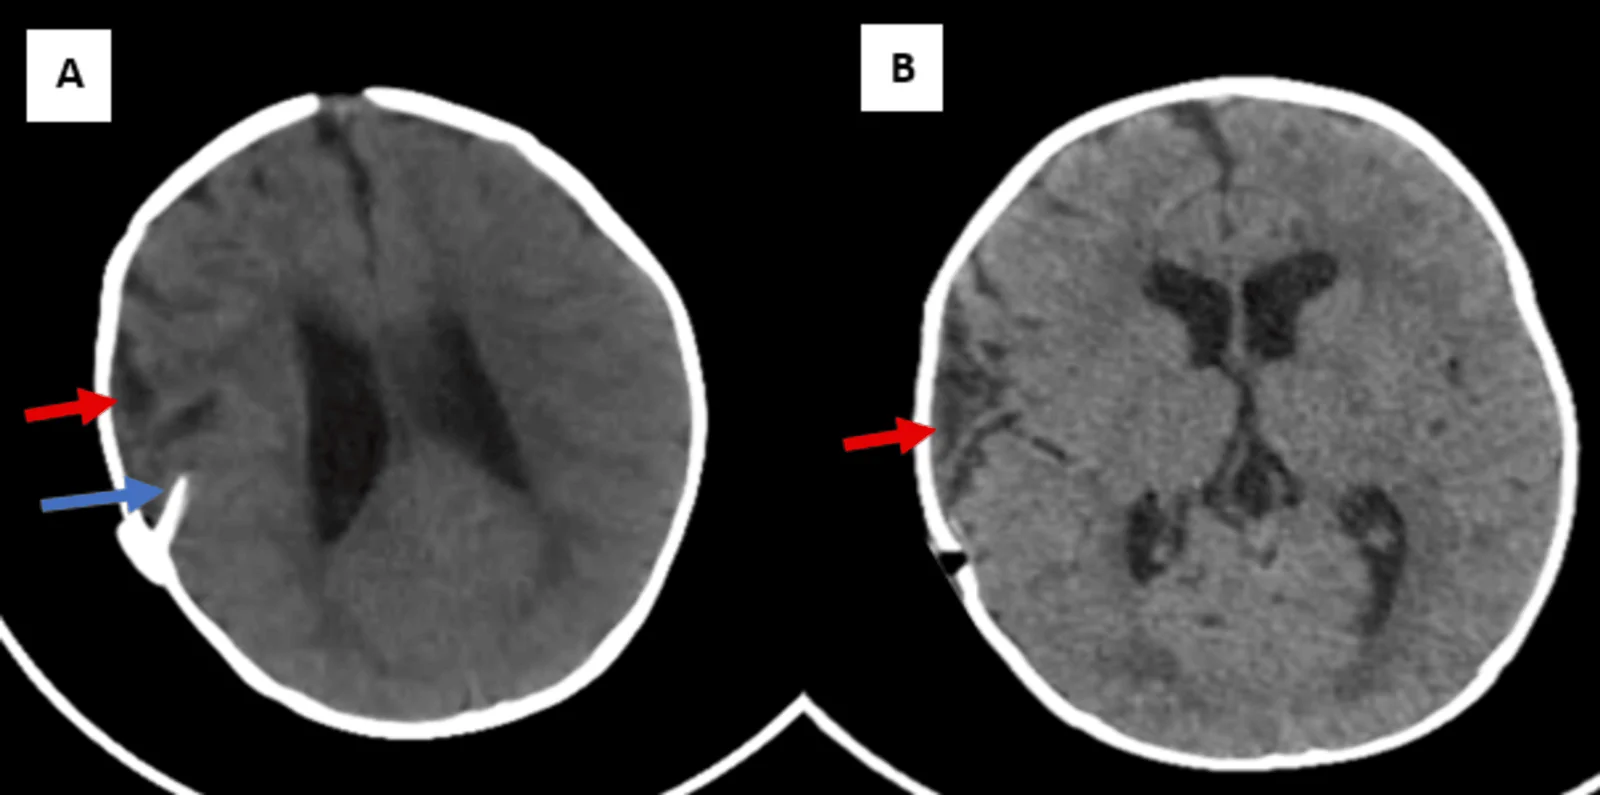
(A) Preoperative brain CT scan obtained after transanal extrusion of the cystoperitoneal shunt, demonstrating the intracranial catheter remaining in its original position (blue arrow) and complete radiological resolution of the arachnoid cyst (red arrow). (B) Follow-up brain CT scan obtained one year after shunt removal, confirming persistent complete resolution of the arachnoid cyst (red arrow) without evidence of recurrence.
